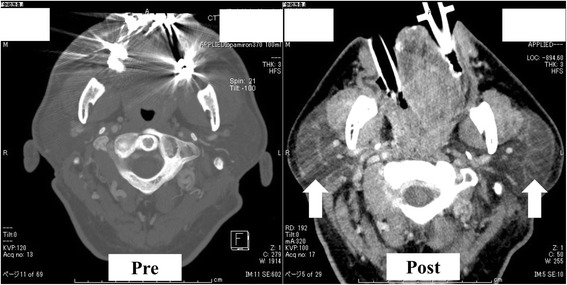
Post-operative image of computed tomography scans at the parotid glands level shows the bilateral parotid glands were swollen compared to the pre-operative state
